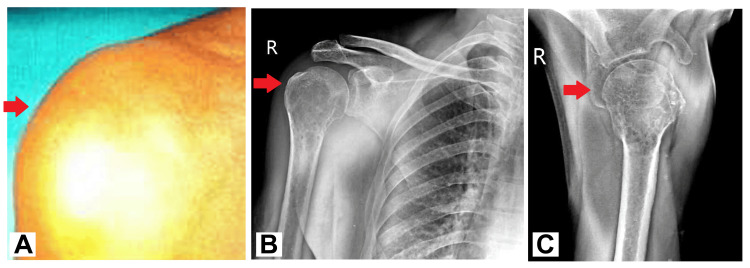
(A) Clinical photographs. (B-C) X-ray of the right shoulder with arm AP and lateral view showing the homogenous radio-opaque area in the proximal end of the humerus AP: anteroposterior
